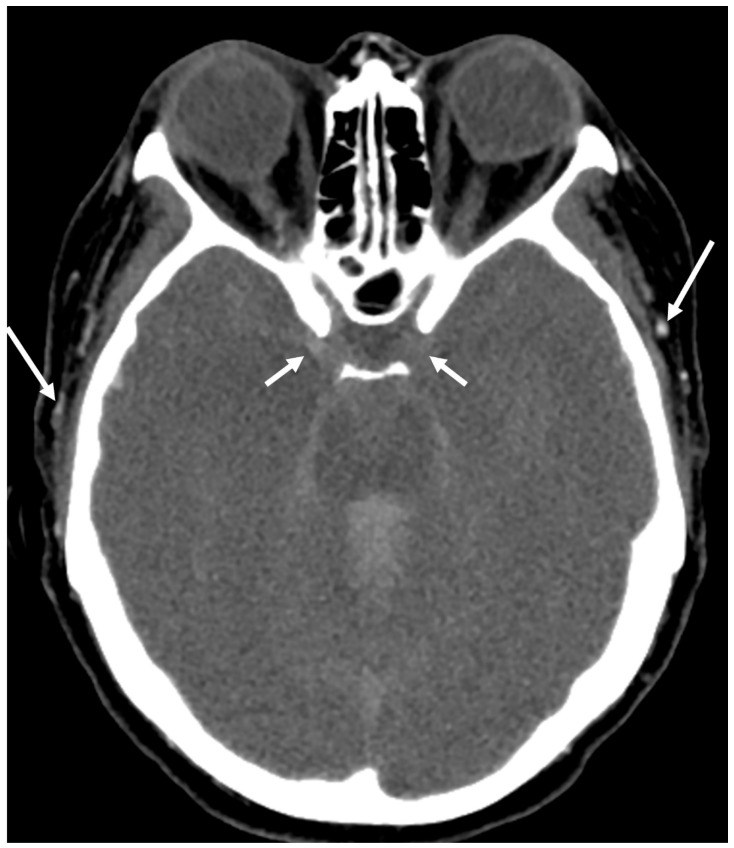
CTA showing classic imaging findings for confirmation of DNC with no opacification of intracranial internal carotid arteries (short arrows) and continued opacification of external carotid branches (long arrows).
